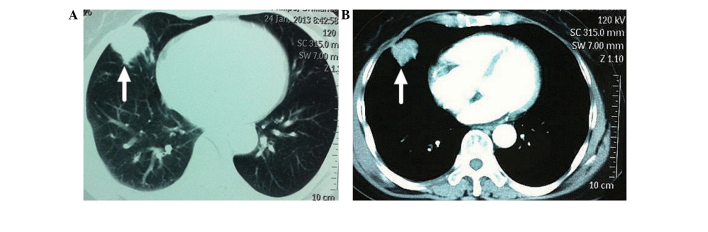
Computed tomography (CT) scans revealing a tissue mass. Enhancement thoracic CT demonstrated a mass in (A) the middle lobe of the right lung and the (B) mediastinum window, which was a possible lung tumor.
